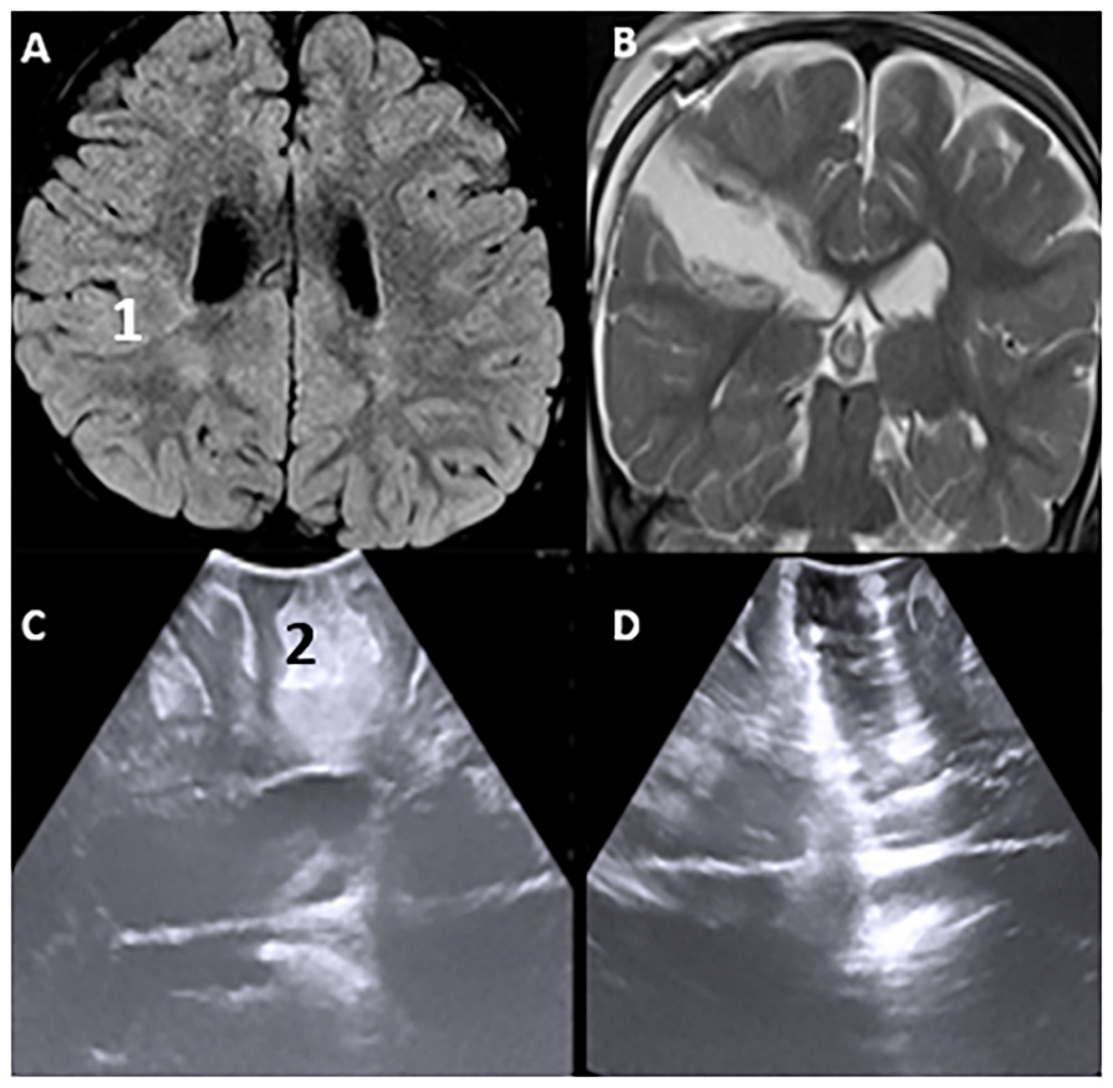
Pre-operative Hyperintence axil MRI FLAIR rt FCD type IIb (A,1) in concordance with pre-resection intraoperative hyper echoic cortical and subcortical IUS features (C,2). Post-operative coronal T2WI (B) and intraoperative post-resection IUS showing complete resection of the lesion (D).
